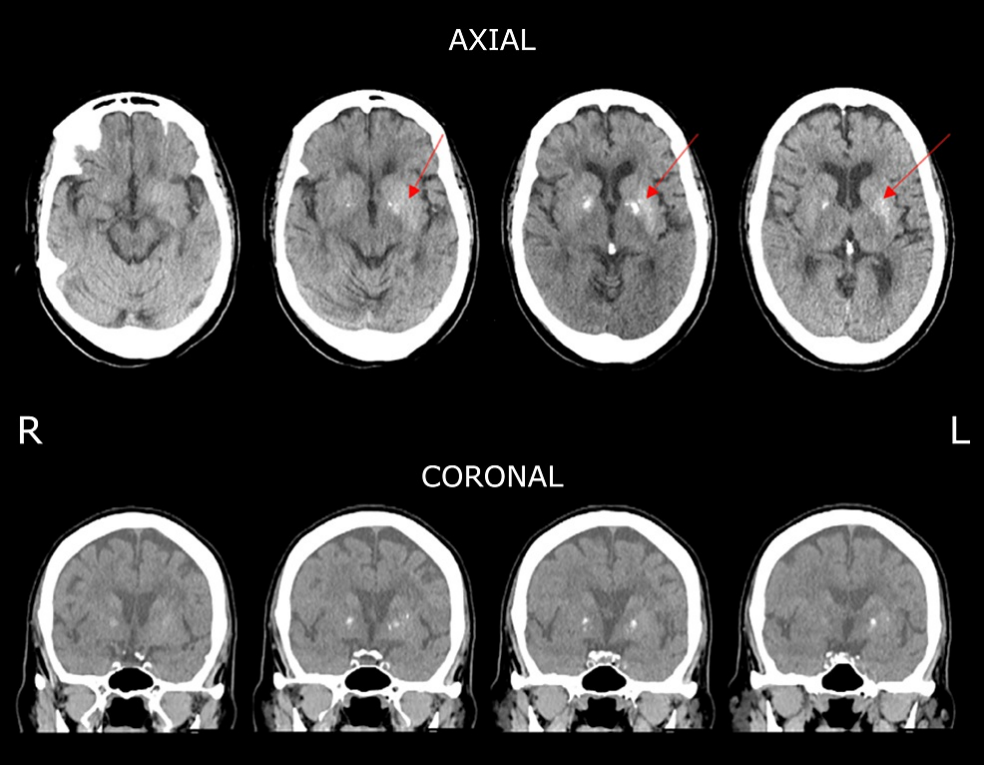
Noncontrast CT of the head showing bilateral basal ganglia calcification with hyperattenuation of the left putamen (red arrows)
